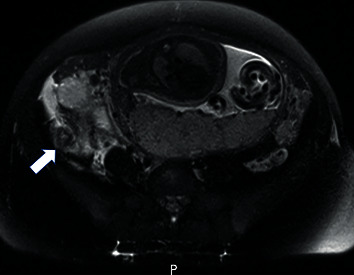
Acute appendicitis detected on T2-weighted MRI image. Dilated appendix (arrow) in the right lower quadrant with surrounding inflammatory changes.
